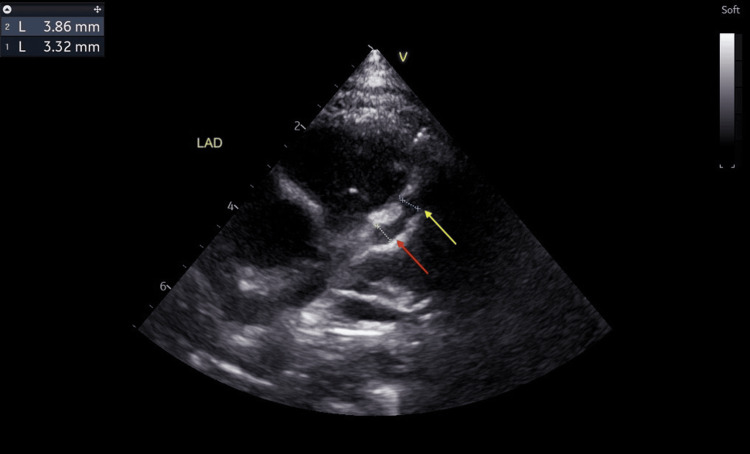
Echocardiogram showing two small fusiform dilatations in the left anterior descending artery. The yellow arrow points at a dilatation measuring 3.32 mm (Z score: +6.16), and the red arrow points at another dilatation measuring 3.86 mm (Z score: +7.84).
